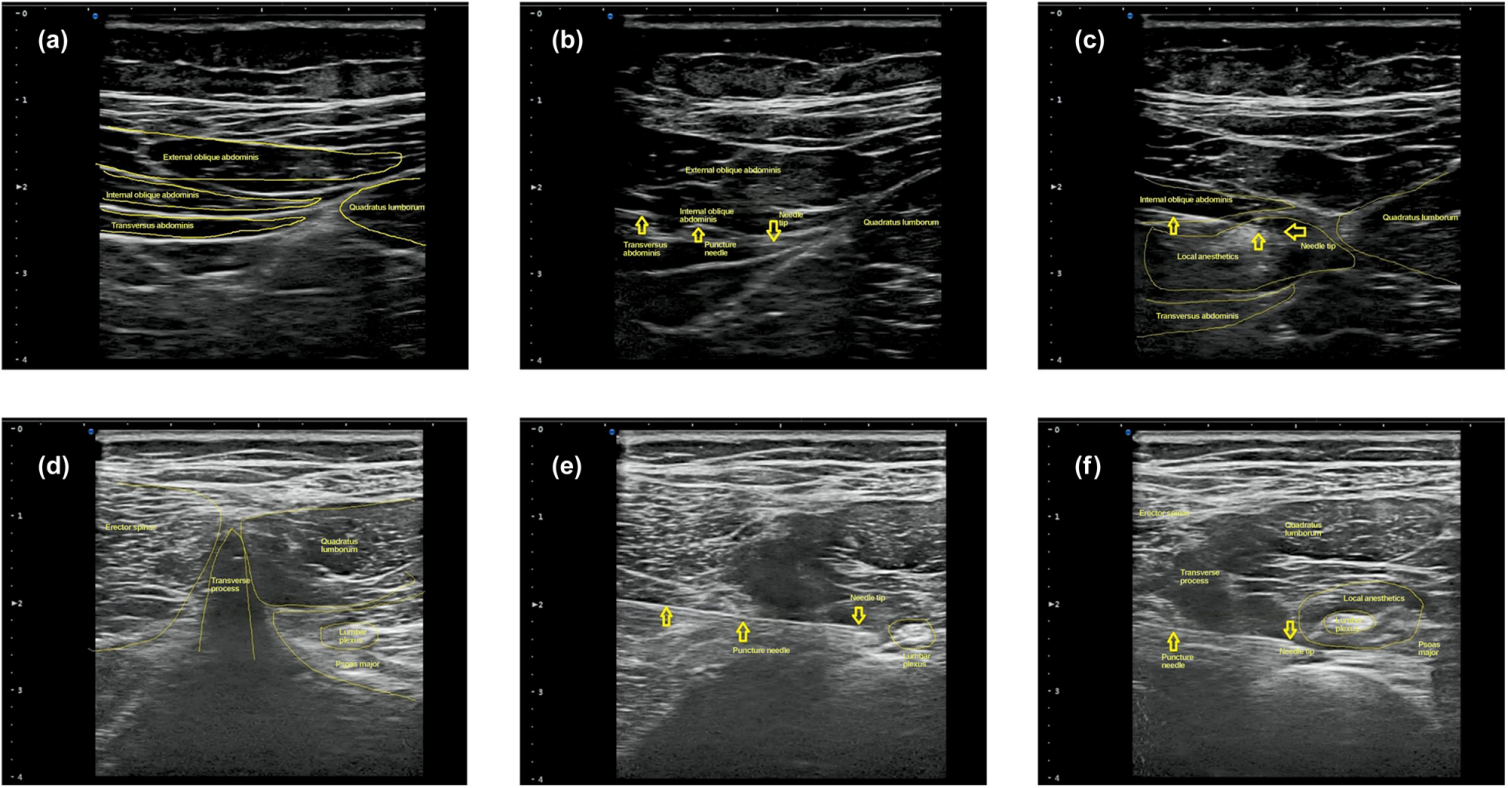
Ultrasound images during local anesthesia. (a–c) Transversus abdominis plane block group (group T). (d–f) Lumbar plexus block group (group L). a/d, b/e, and c/f are representative images for positioning, puncture, and 3 min after drug injection, respectively. The yellow arrows show the position of puncture needles.
